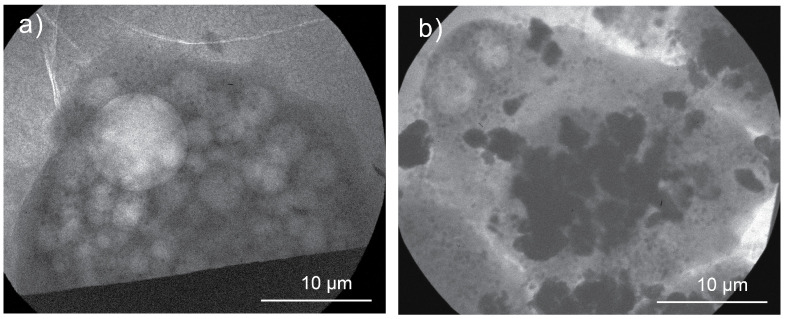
X-ray macrographs of amoebas exposed to ${\mathrm{MoO}}_{2}$ NPs. (a) Control cell. (b) Amoeba exposed to ${\mathrm{MoO}}_{2}$ NPs for 4 h. The samples were prepared with the optimized system.
